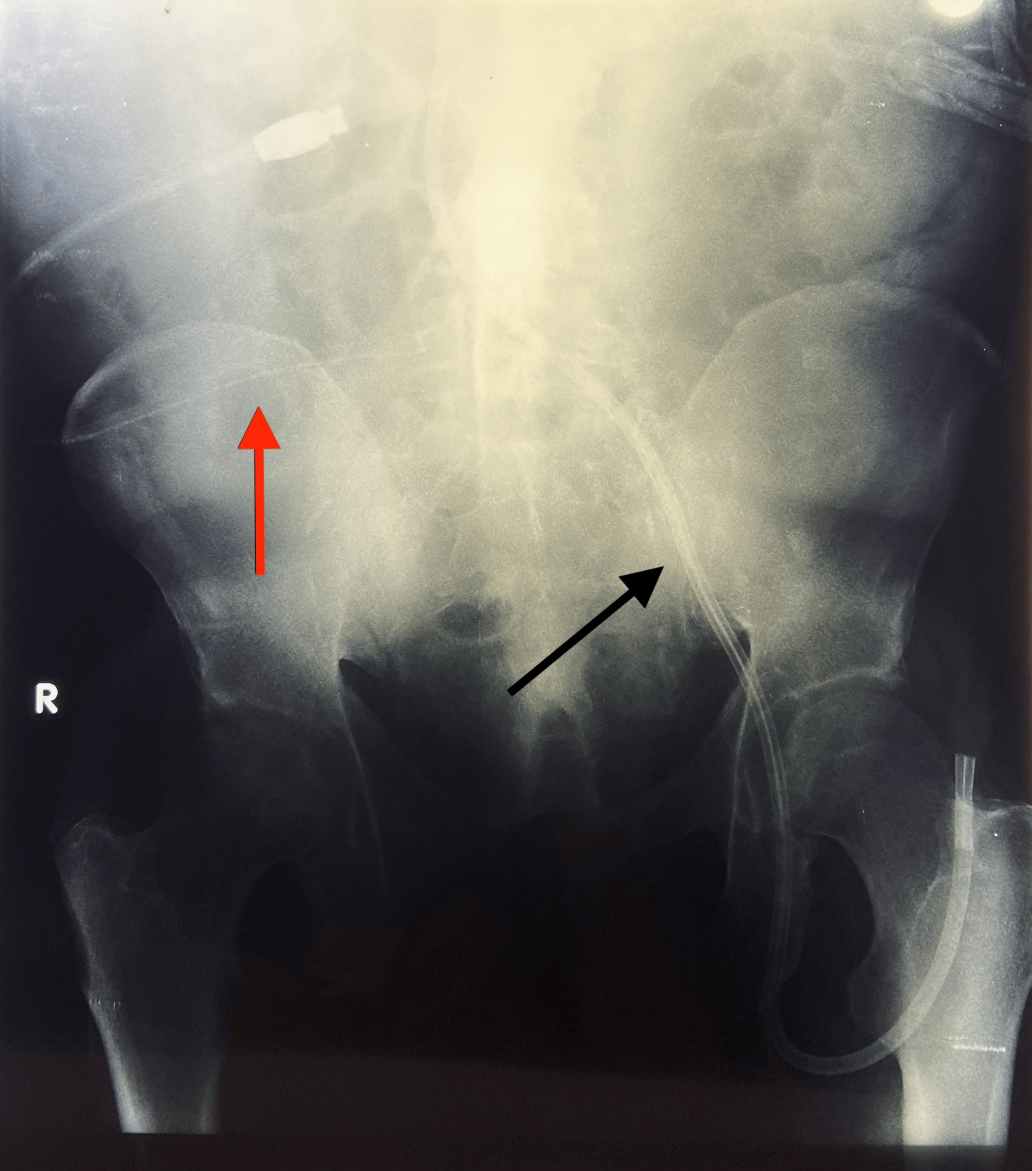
Native X-ray of the abdomen showing the PC (red arrow) and infected Hickman CVC (black arrow). PC: Peritoneal catheter; CVC: Central venous catheter.
